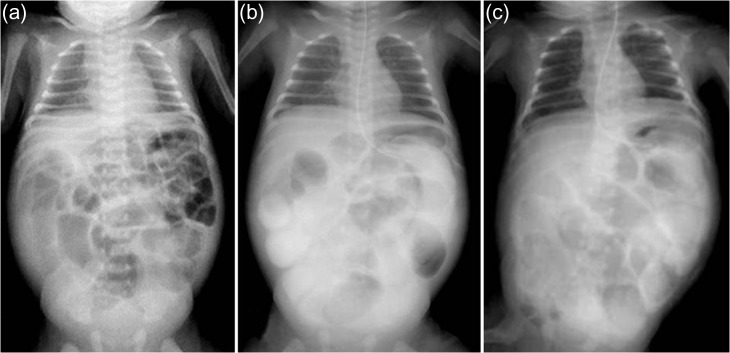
Abdominal radiography. (a) Admission films show partially dilated loops of bowel with intestinal gas (age 20 days). (b and c) Time progression radiography. Five hours after injecting contrast through a gastric tube (b, age 23 days) and 16 h after injecting contrast medium (c, age 24 days); dilated loops of bowel with intestinal gas are visible, with residual contrast medium.
